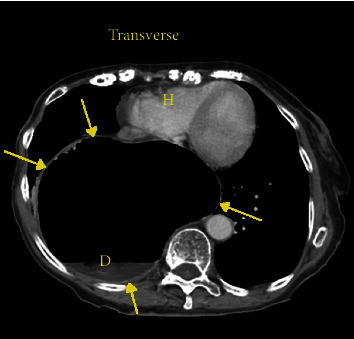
Transverse chest CT of the abdomen showing large hiatal hernia with concern for gastric volvulus or gastric outlet obstruction. The large hernia is between the yellow arrows. The heart is “H,” and the debris in the bowel is “D.”
